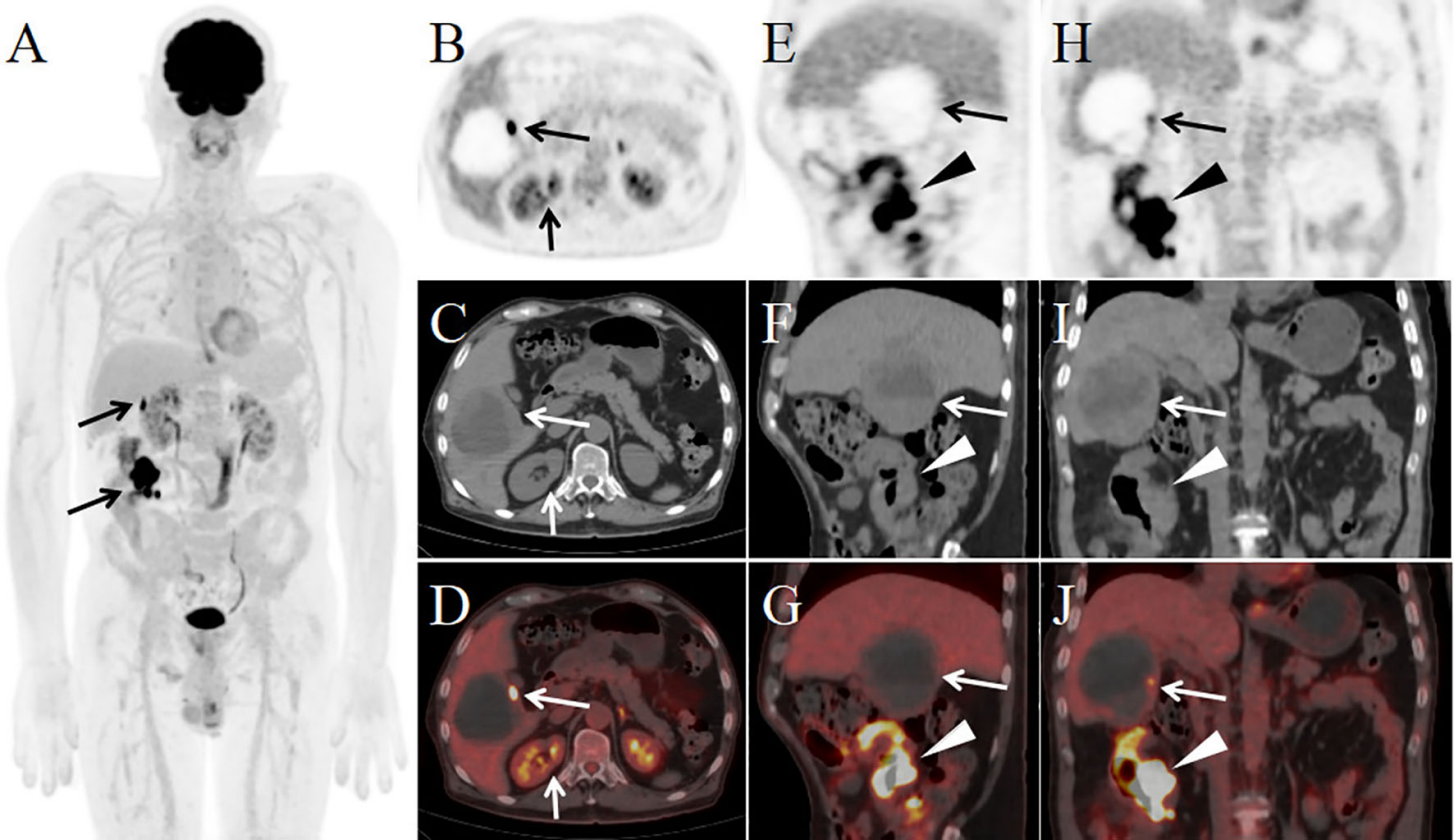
PET/CT images after four cycles of chemotherapy [(A) MIP; (B, E, H) PET; (C, F, I) CT; (D, G, J) PET/CT]. The MIP image (A) reveals nodular and patchy areas of abnormally increased FDG uptake in the liver and right abdomen. Axial (B–D), sagittal (E–G), and coronal (H–J) PET/CT images demonstrate a hepatic lesion (B–J, long arrows) measuring 7.3 cm × 7.6 cm × 7.9 cm, with nodular areas of increased FDG uptake at the lesion margins (SUVmax: 13.9), while FDG uptake is absent in the remaining portions of the lesion. The ileal lesion (E–J, arrowheads) shows a markedly reduced extent compared to baseline, with a maximum wall thickness of 2.1 cm and abnormally increased FDG uptake (SUVmax: 21.2). The previously noted right renal lesion (B–D, short arrows) has completely resolved, with no abnormal FDG uptake observed.
